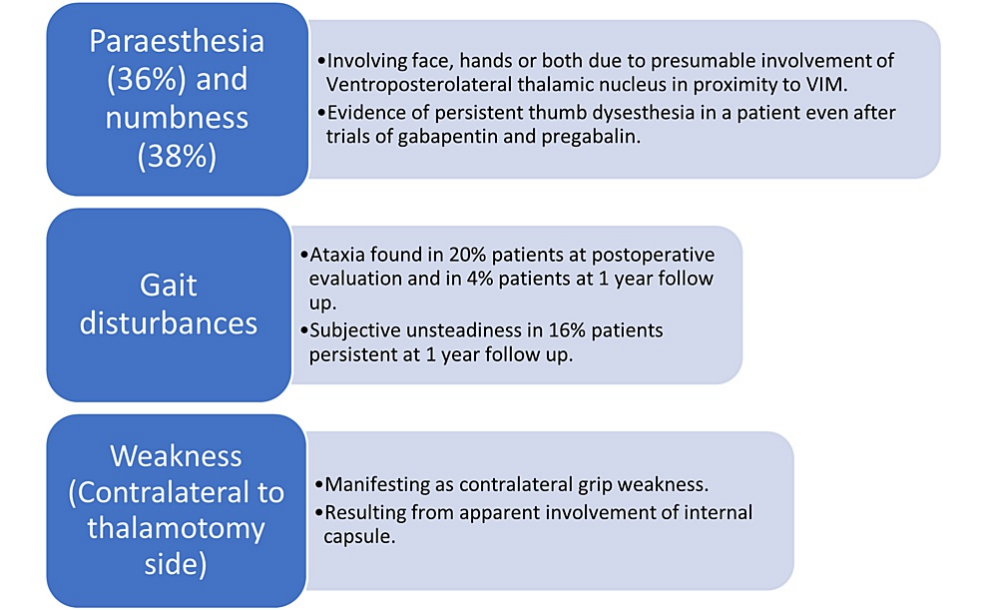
Side-effect profile of magnetic resonance imaging-guided focused ultrasound sonication
